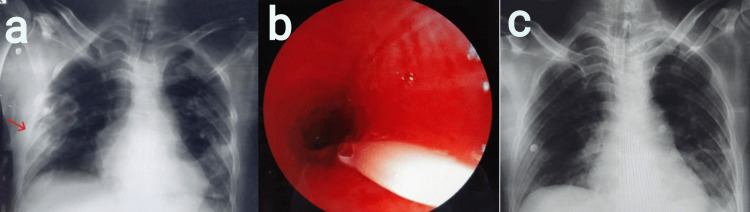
a) Chest radiograph revealed the segmental collapse of the right lower lobe, b) flexible bronchoscopy revealed mucus plug in the superior segment of the right lower lobe bronchus, and c) chest X-ray revealed the resolution of atelectasis following the removal of mucus plugs
